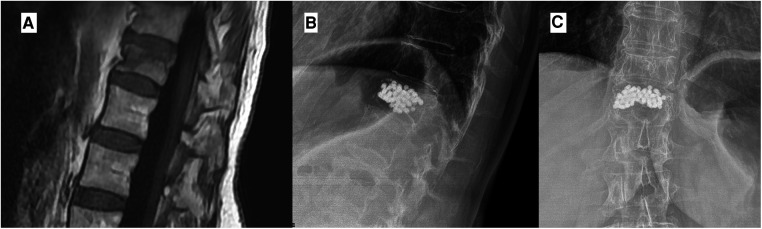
In this case, a 76-year-old woman with vertebral compression active fracture visible in MRI with STIR sequences (A) was treated with D11 vertebroplasty with the injection of titanium microspheres. The follow-up radiographic control shows the stability of the implant without any sign of leakage (B,C).
